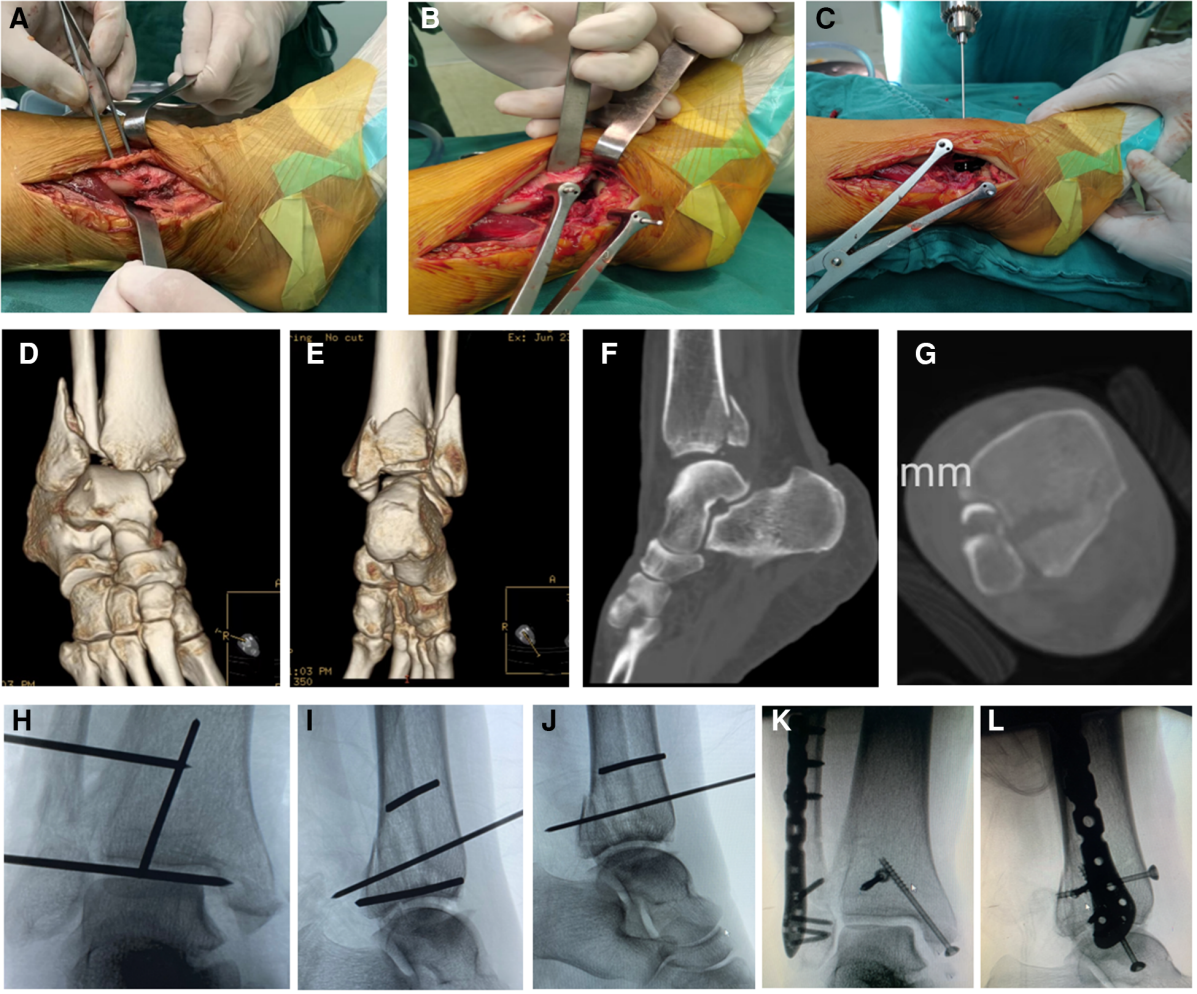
Surgical procedure of a single lateral approach *via* stretching fibular fracture line. (A) A single lateral incision was used to expose the broken end of the fibula fracture. (B) The ankle joint was distracted with a Hintermann retractor to obtain sufficient working space. (C) A hollow guide needle was inserted from the anterior tibia to the posterolateral tibia to fix the posterior malleolus fracture. (D,E) Preoperative 3D reconstruction images. (F) Preoperative sagittal CT image. (G) Preoperative transverse CT image. (H) Intraoperative anterior–posterior x-ray image showed the fixation of the fracture with Kirschner wires. (I) Intraoperative lateral x-ray image showed the fixation of the posterior malleolus fracture with the Kirschner wire and the fracture reduction was mildly poor. (J) After adjustment, anatomic reduction with the Kirschner wire. (K) Postoperative anterior–posterior x-ray image. (L) Postoperative lateral x-ray image.
